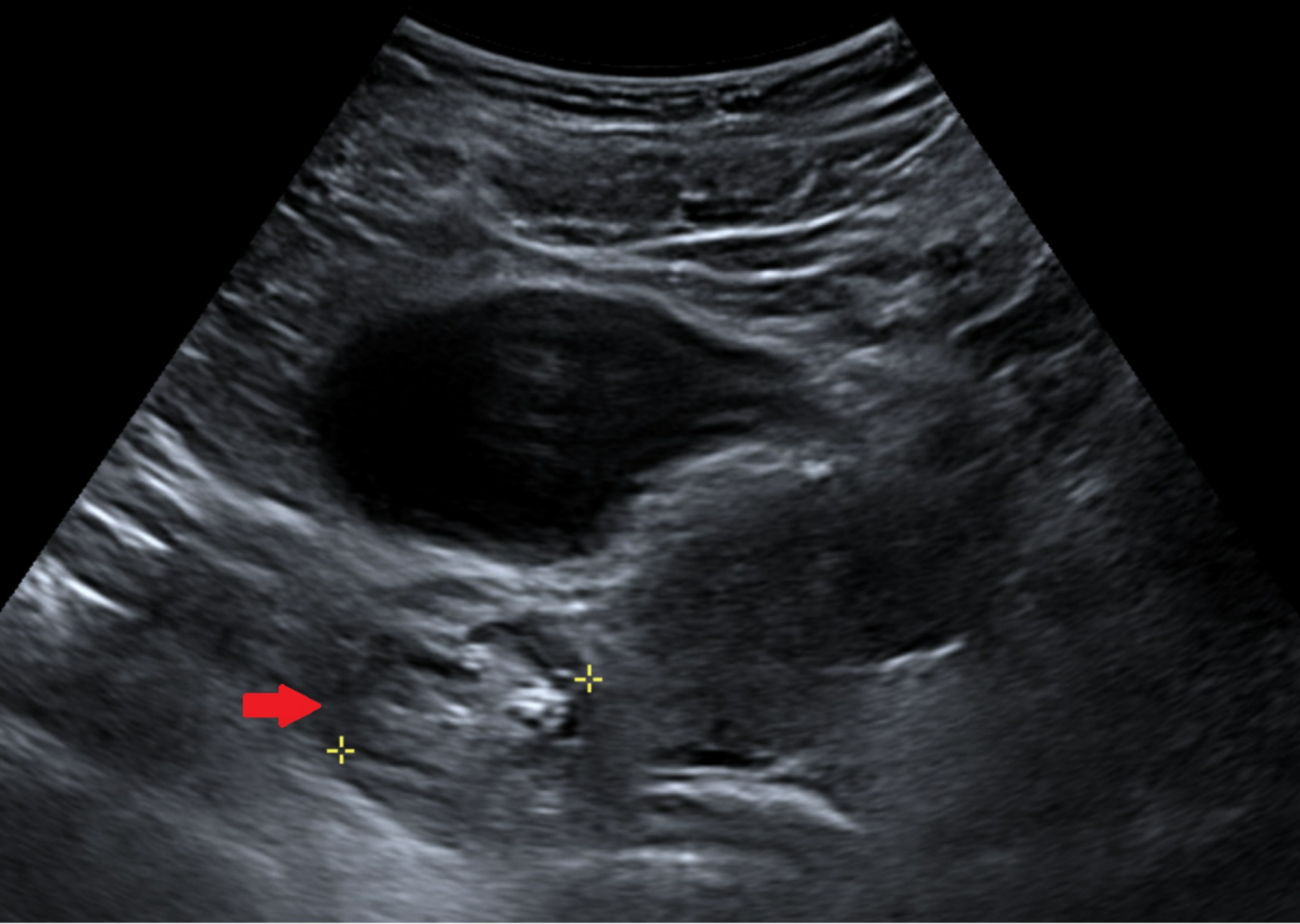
Transabdominal pelvic ultrasound Red arrow showing a solid mass in the right ovary.
